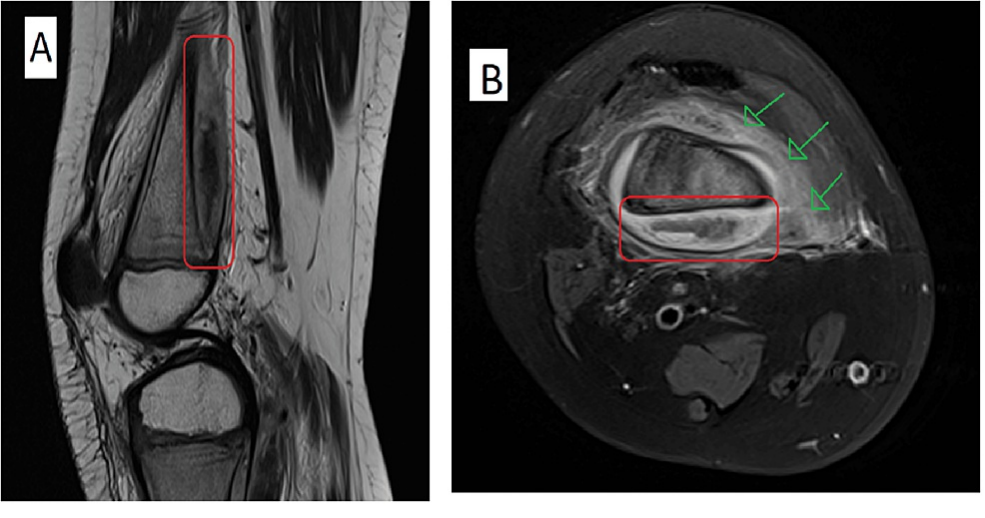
MRI RT knee. (A, B) Distal femur subperiosteal collection (red rectangular). (B) Femur surrounding soft tissue edema (green arrows). RT: right.
